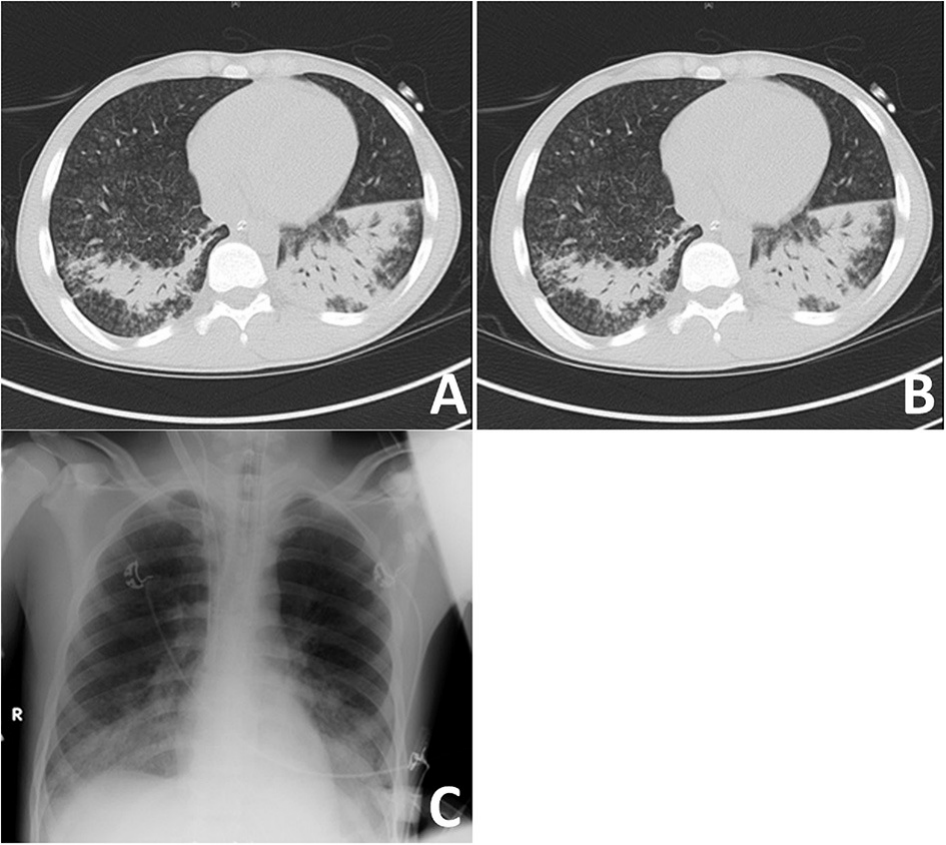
Imaging of most severe autoimmune lung disease on admission. (A) Recruitment CT-scan for acute respiratory distress syndrome (ARDS) at FiO_2_ of 100% in deep analgo-sedation, as well as muscle relaxation with cis-atracurium at a PEEP-level of 5 cmH_2_O and PIP at 15 cmH_2_O with tidal volumes at 6 ml/kg ideal body weight (IBW) in an expiratory hold after 3 min at this level; (B) CT-scan after 1 min of recruitment at a PEEP-level of 10 cm H_2_0 and a PIP of 35 cm H_2_O, followed by 1 min at a PEEP-level of 20 cm H_2_O and a PIP of 45 cm H_2_O at an inspiratory hold; (C) Conventional imaging with bilateral inflammatory consolidations in lower lobes.
